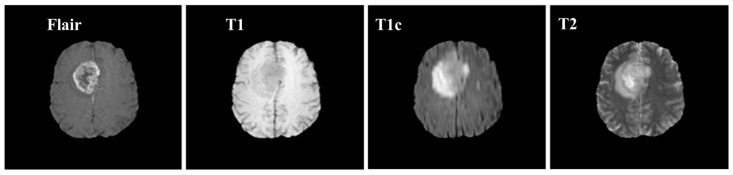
Sample MR images of T1, T2, T1c and Flair modalities.
